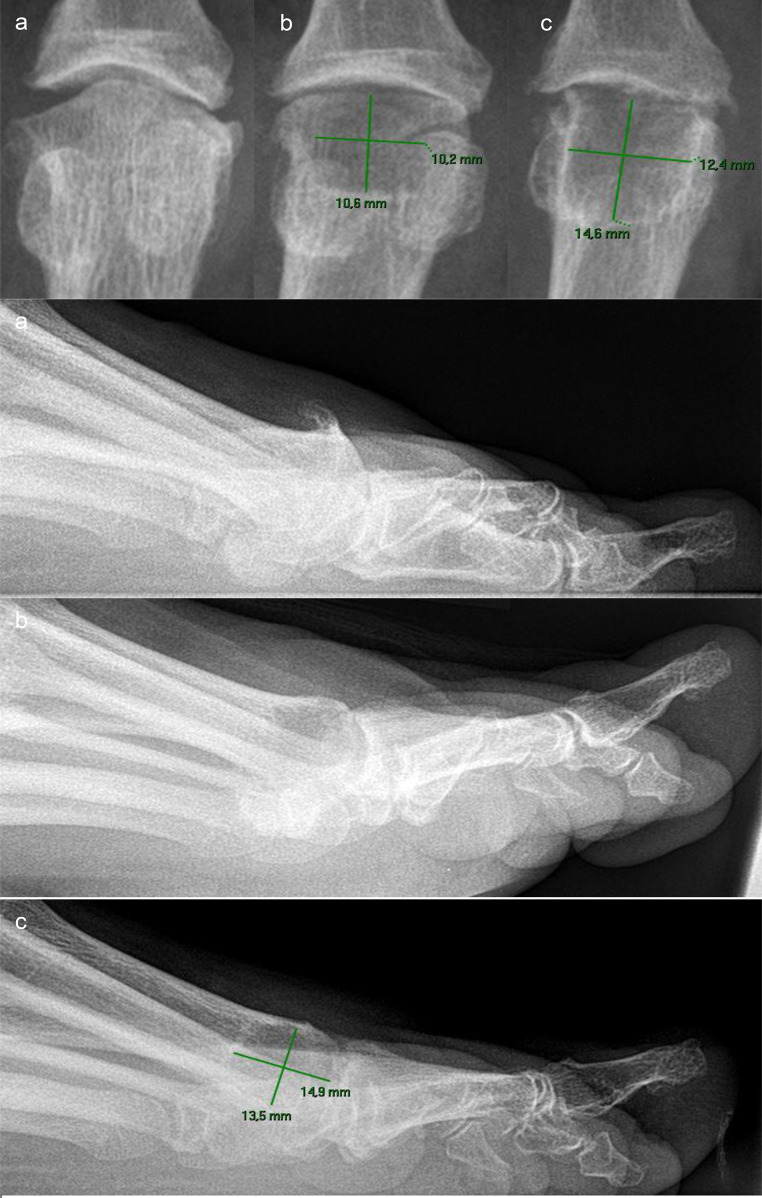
Severe case of osteolytic expansion of the implant site a: Preoperative condition. b: Radiographic control 2 days postoperatively: correct location of the bone canal of adequate size for a 10 × 10 mm Cartiva SCI. c: Follow-up, 13 months postoperatively: Extensive enlargement of the implant site to 12.4 × 14.6 mm in anteroposterior projection respectively 13.5 × 14.9 mm in lateral projection. Additionally: bright sclerotic margin, severe erosive changes on the bone surrounding the implant and the articular surface of the proximal phalanx
